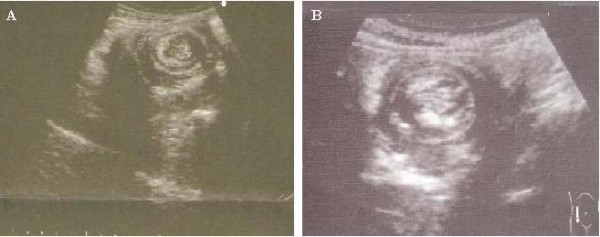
Transverse sonographic image of the intussusception. Note the hypoechoic outer layer of edematous bowel wall with echogenic layers, known as the "bull's-eye" or "target" signs.
